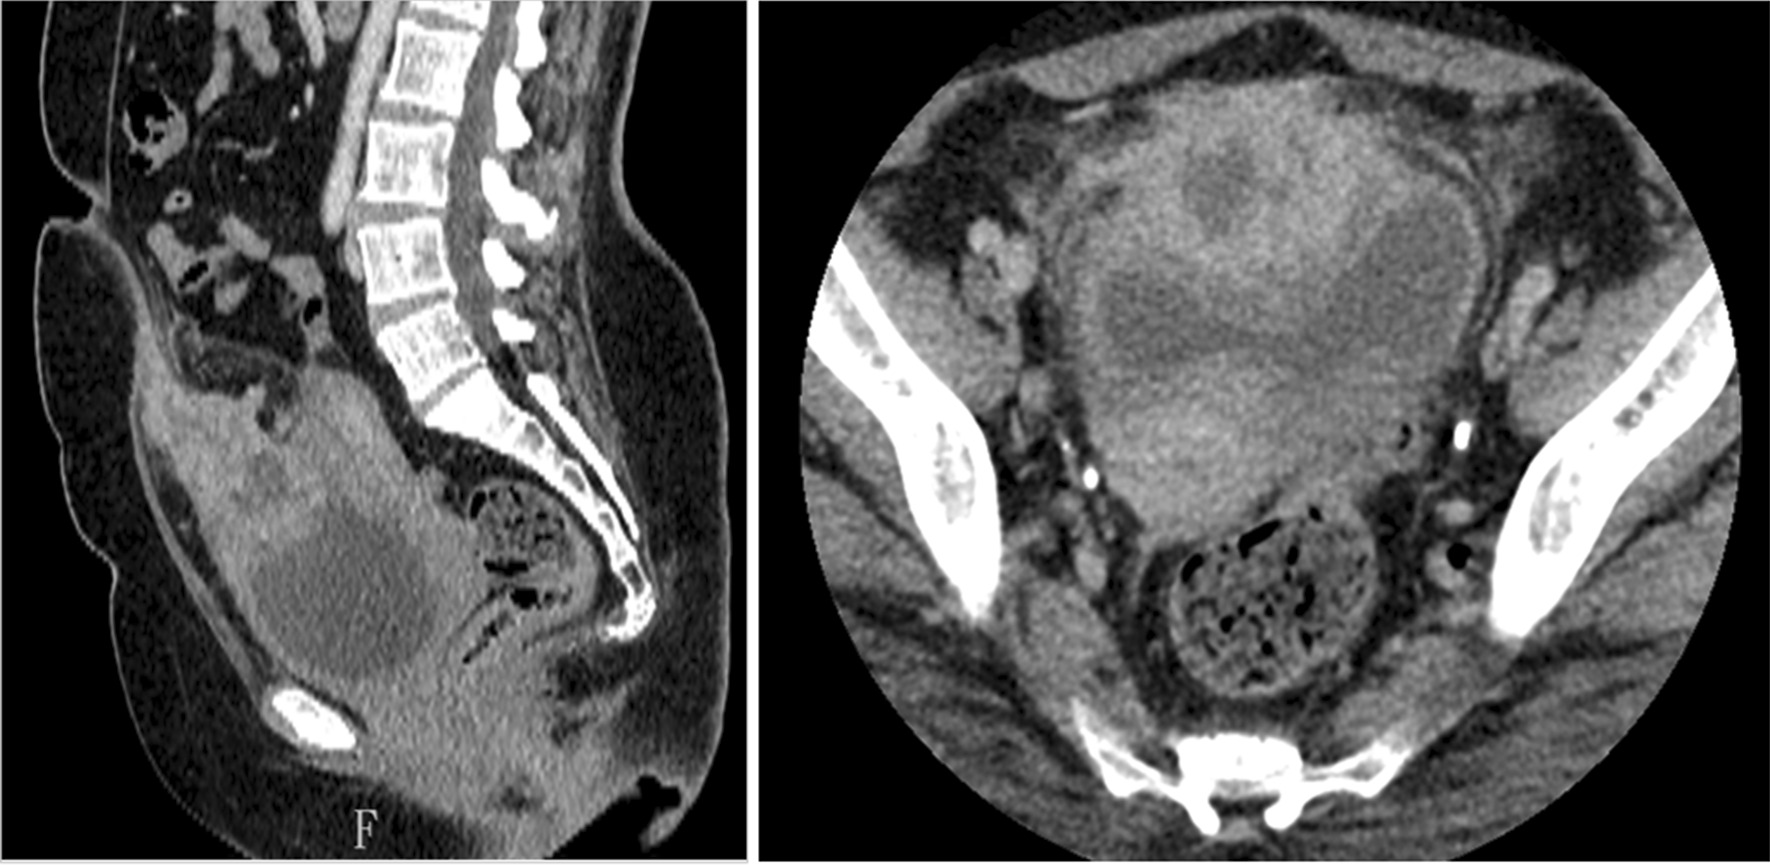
Abdominal CT showing a midline suprapubic mass of heterogeneous enhancement between umbilicus and bladder. The mass consisted of low attenuation cystic element surrounded by thick, enhancing wall
